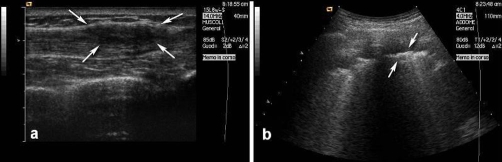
Chest wall ultrasounds showing an ill-defined subcutaneous lesion with inhomogeneous hypoechoic structure (a). Hyperechoic linear thickening of pleural surface consistent with calcified pleural plaques are seen (b).
